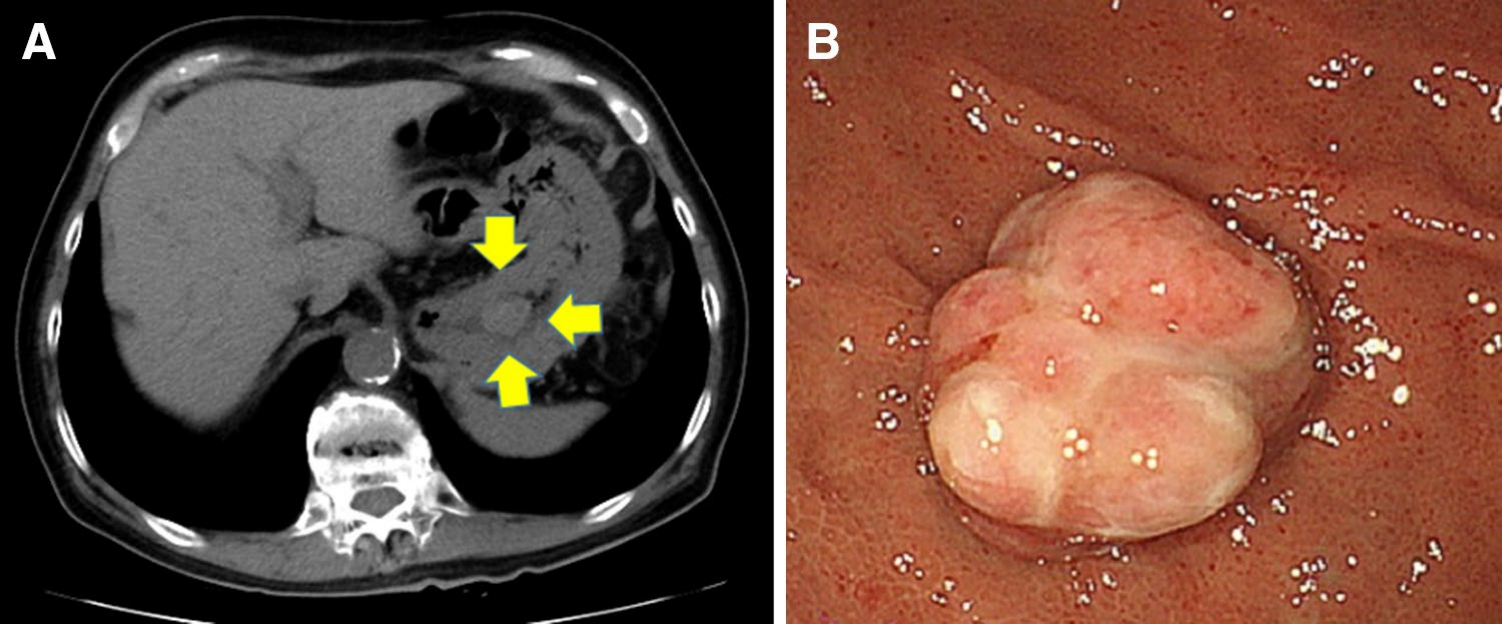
Gastric metastasis from renal cell cancer. Routine follow-up CT scan revealed a tumor lesion in the stomach (a). Endoscopic appearance of a solitary, distinct polypoid lesion (b)
